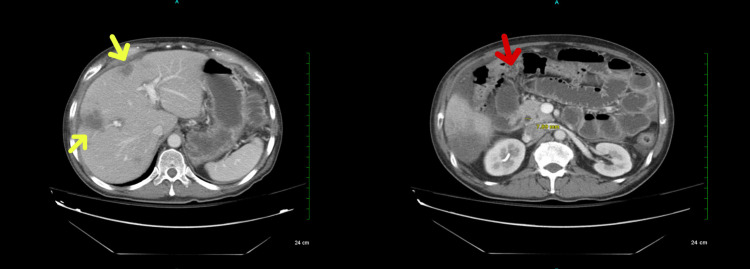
CT scan of abdomen and pelvis, showing numerous metastatic liver lesions (yellow arrows) and small bowel obstruction (red arrow).
